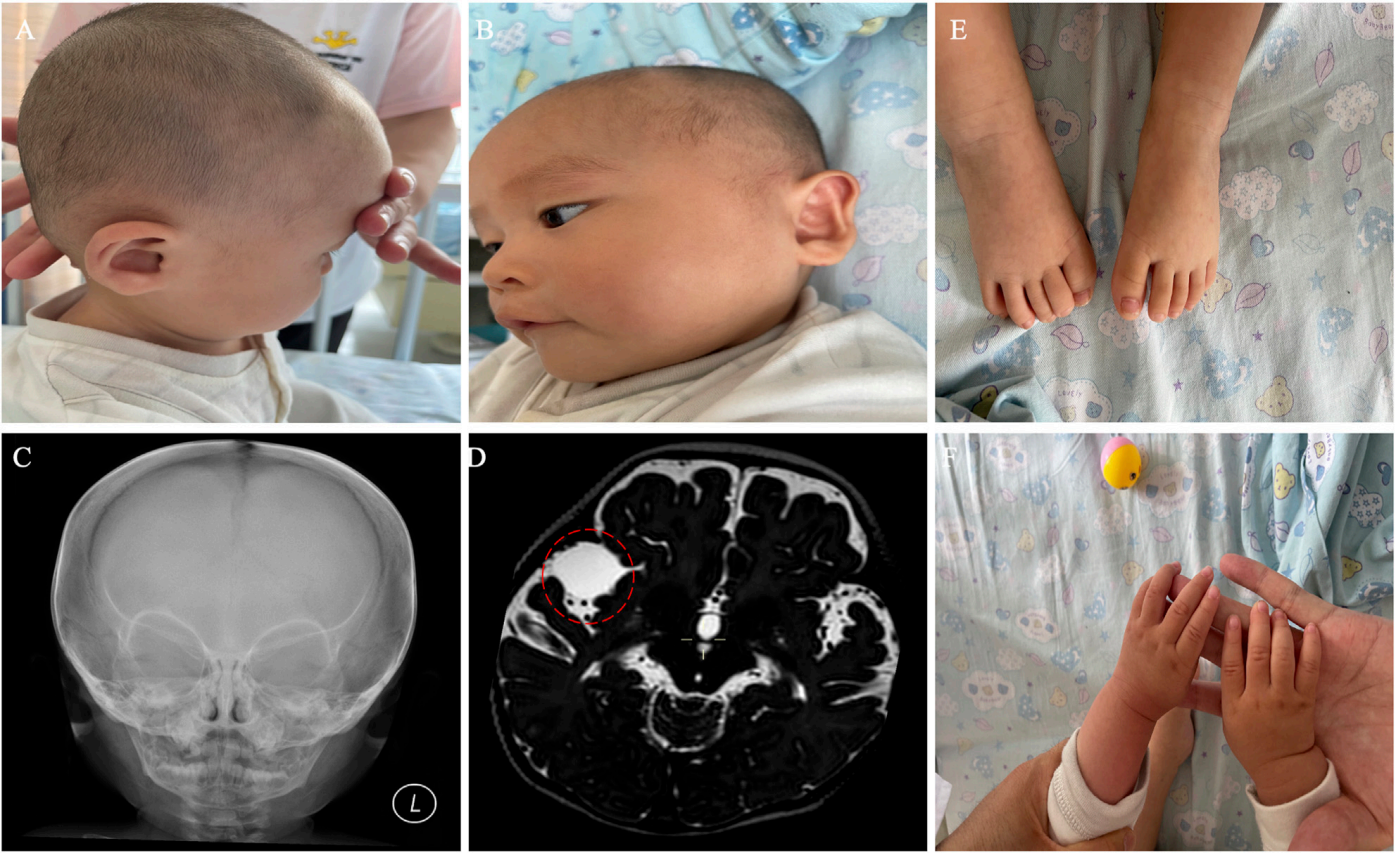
Clinical phenotypes of the proband with 7p22.3 deletion and 7q36.3 duplication at 10 months of age. (A,B) Dysmorphic facial features of the proband. (C) X-ray of the face. (D) Brain MRI showed a middle cranial fossa meningioma. The dashed red circle marks the approximate boundaries of the region associated with a middle cranial fossa meningioma. (E,F) normal trunk and limbs.
